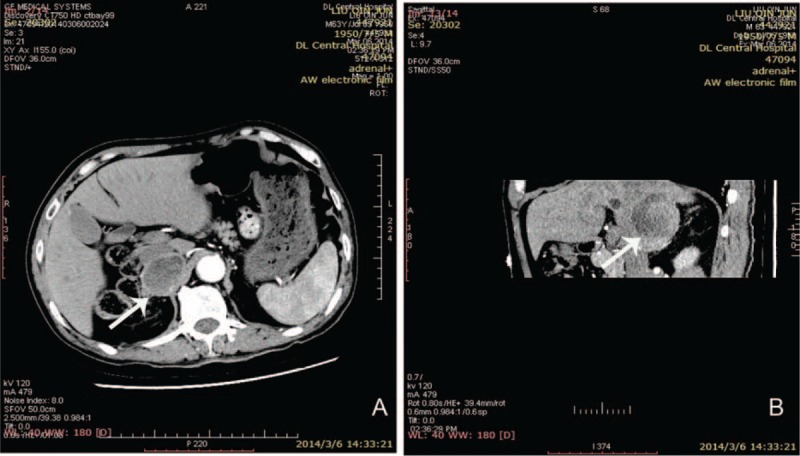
Abdominal computed tomography scan (arterial phase) a tumor in right adrenal gland accompanied.
